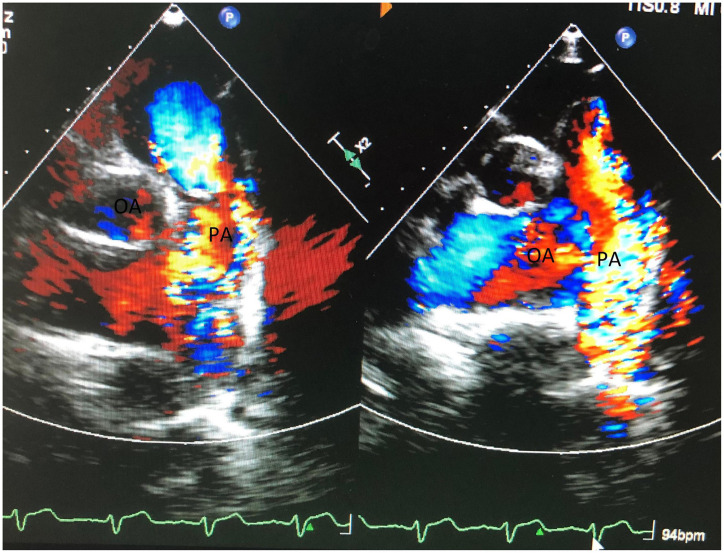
Shows obvious high velocity continuous and dominant diastolic aliasing color flow Doppler signals in MPA.
